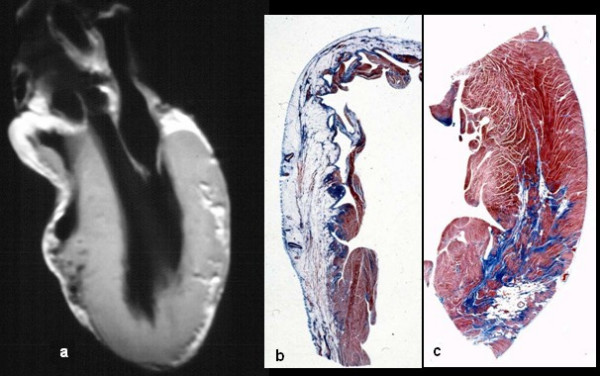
Same case of fig. 2. Note the biventricular involvement at long axis *in vitro *MRI (a), with transmural fibro-fatty replacement in the RV free wall (b) and focal subepicardial in the LV free wall (c).
